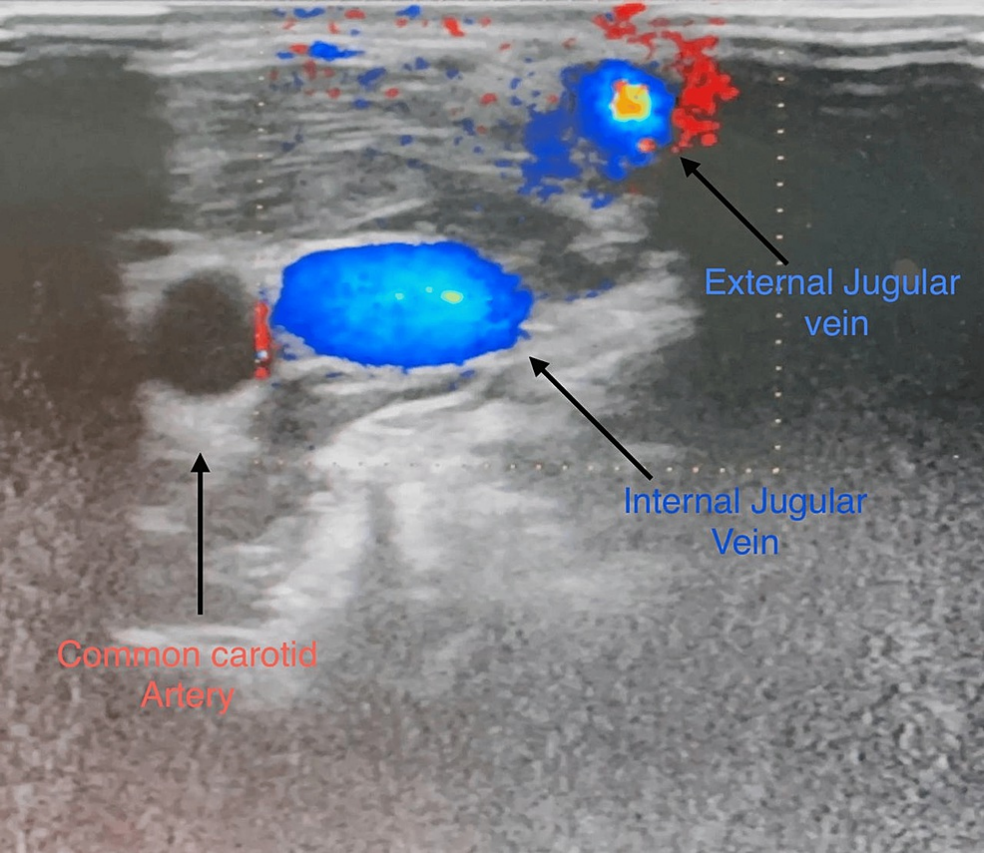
Color Doppler showing slow-flow venous malformation of the right external jugular vein
